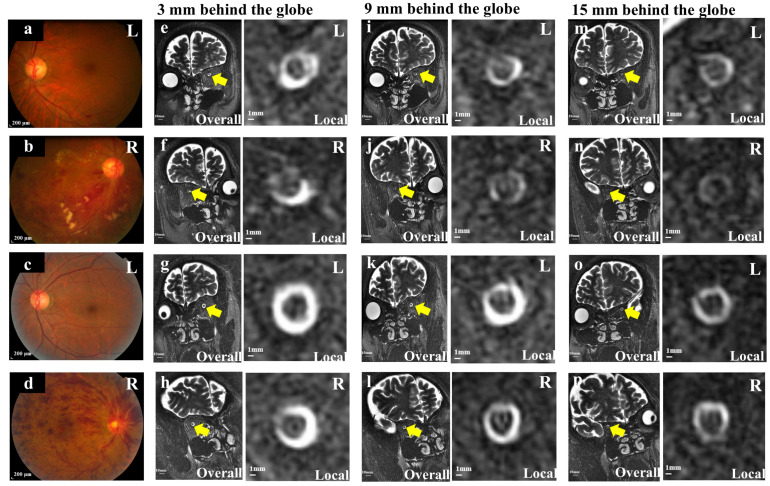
MRI images showed the ONSASW 3 mm, 9 mm, and 15 mm behind the globe in RVO eyes (color photos). (a,b): The color fundus photography of BRVO-affected or contralateral normal eyes. (c,d): The color fundus photography of CRVO-affected or contralateral normal eyes. (e–p) showed an oblique coronal T2WI-FRFSE image with fat suppression to demonstrate the optic nerve sheath complex taken at 3 mm (e–h), 9 mm (i–l), and 15 mm (m–p) behind the globe. (a,b,e,f,i,j,m,n): A 49-year-old man with BRVO and contralateral normal eyes. (c,d,g,h,k,l,o,p): A 51-year-old man with CRVO and contralateral normal eyes. Yellow arrow: ONSASW. BRVO: Branch retinal vein occlusion. CRVO: Central retinal vein occlusion. ONSASW: Optic nerve subarachnoid space width.
